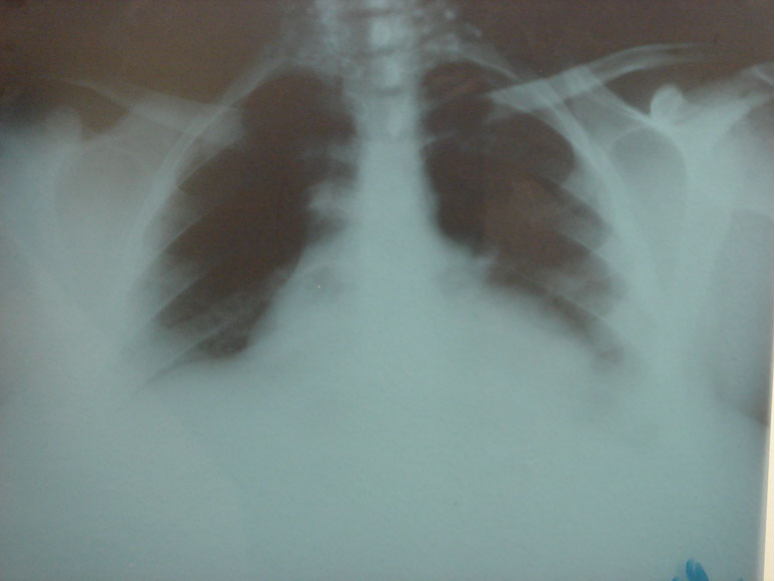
Plain chest X-ray shows no pleural effusion or metastasis. Upward displacement of the diaphragm affecting air entry into the lower lobes of lungs (explains patient's tachypnea).
